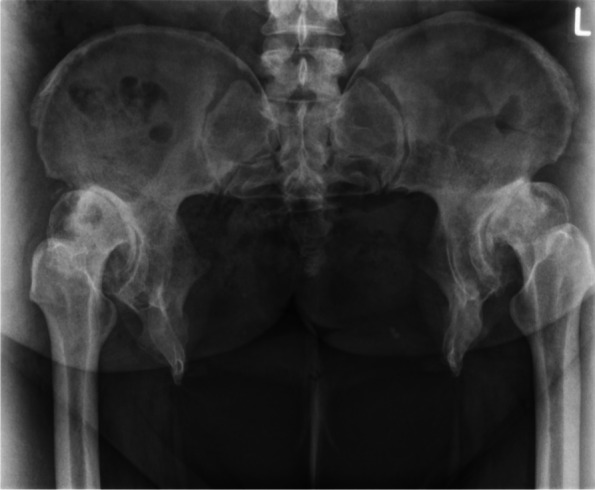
Antero-posterior radiography of the pelvis which shows advanced osteoarthritis secondary to hip dysplasia of both sides combined with a congenital pubic diastasis of 18 cm
